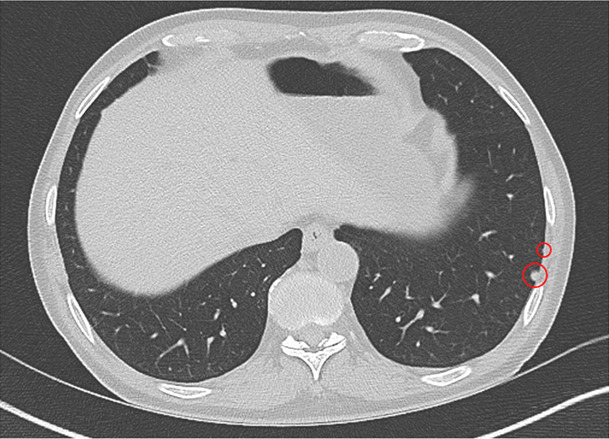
CT chest with long-term and stable subcentimeter pulmonary nodules.
